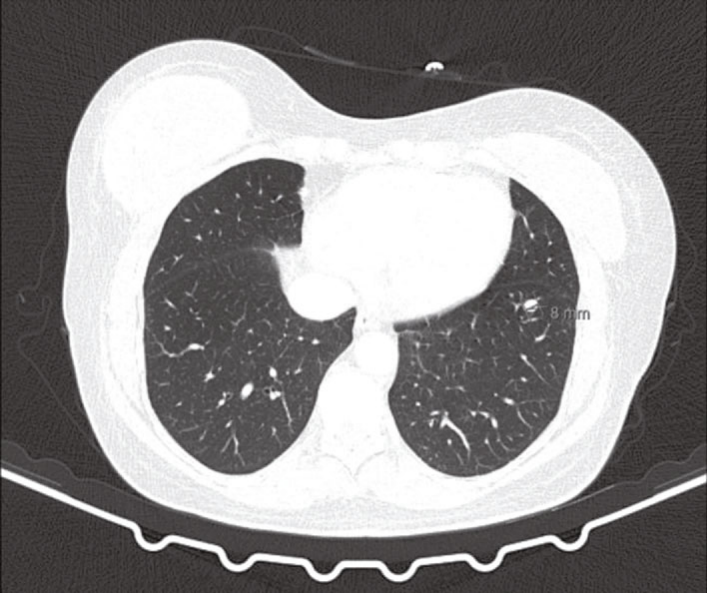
Transverse section of a thorax CT scan presenting an 8 mm pulmonary nodule.
